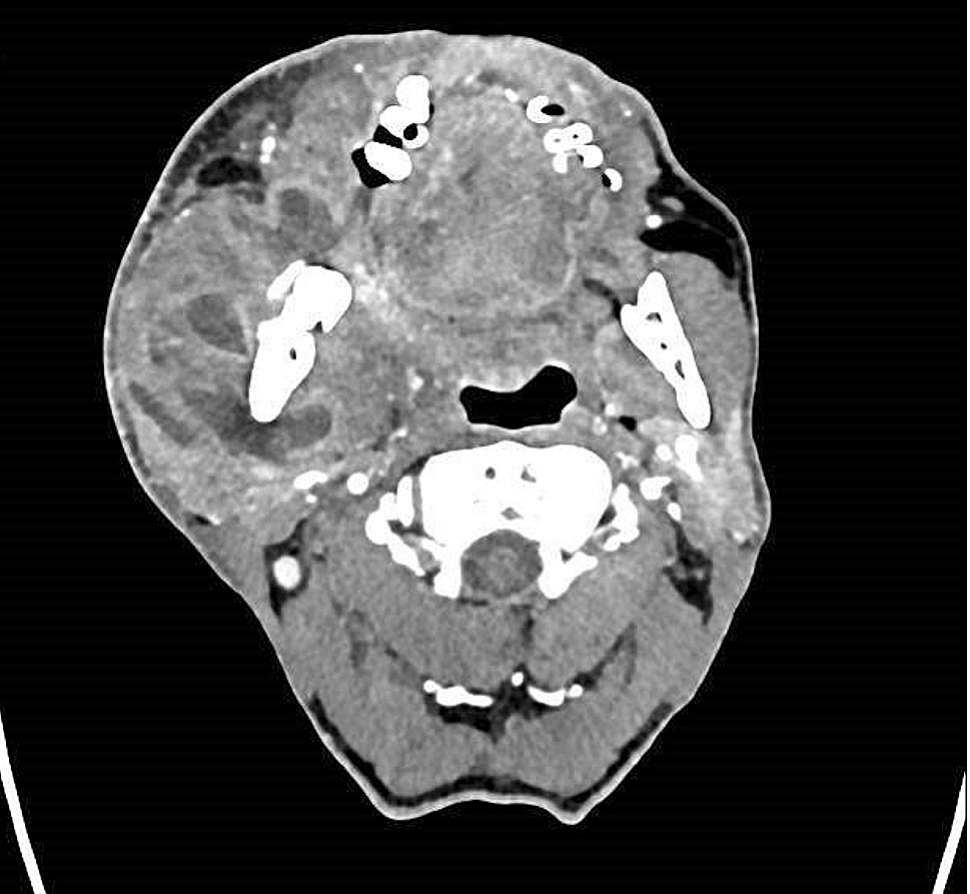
Contrast-enhanced CT scan presenting a multilocular abscess of a middle-aged male patient who has a history of epilepsy and smoking, presented with a few days neck and cheek swelling, trismus and C-reactive-protein reported 305 mg/L at admission
